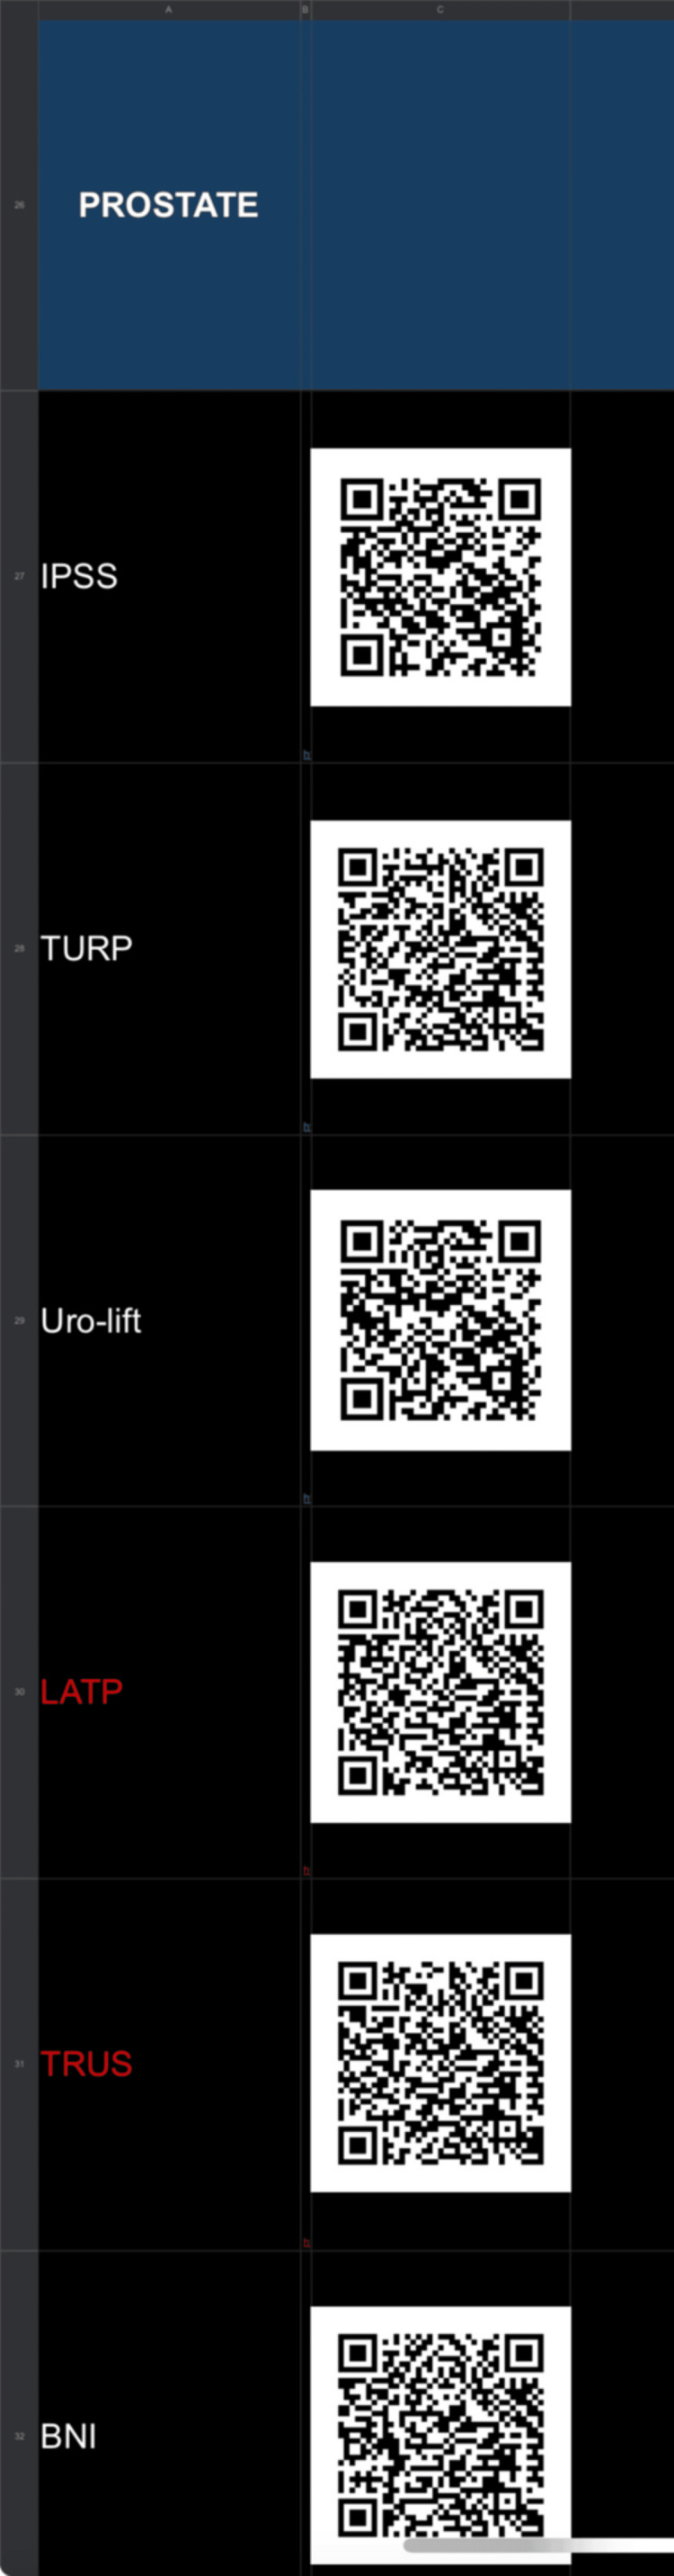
Sample of the author's spreadsheet for PILs related to the prostate PILs: patient information leaflets; IPSS: International prostate symptom score; TURP: transurethral resection of the prostate; Uro-lift: UroLift system; LATP: laparoscopic-assisted transperitoneal prostatectomy; TRUS: transrectal ultrasound; BNI: bladder neck incision
